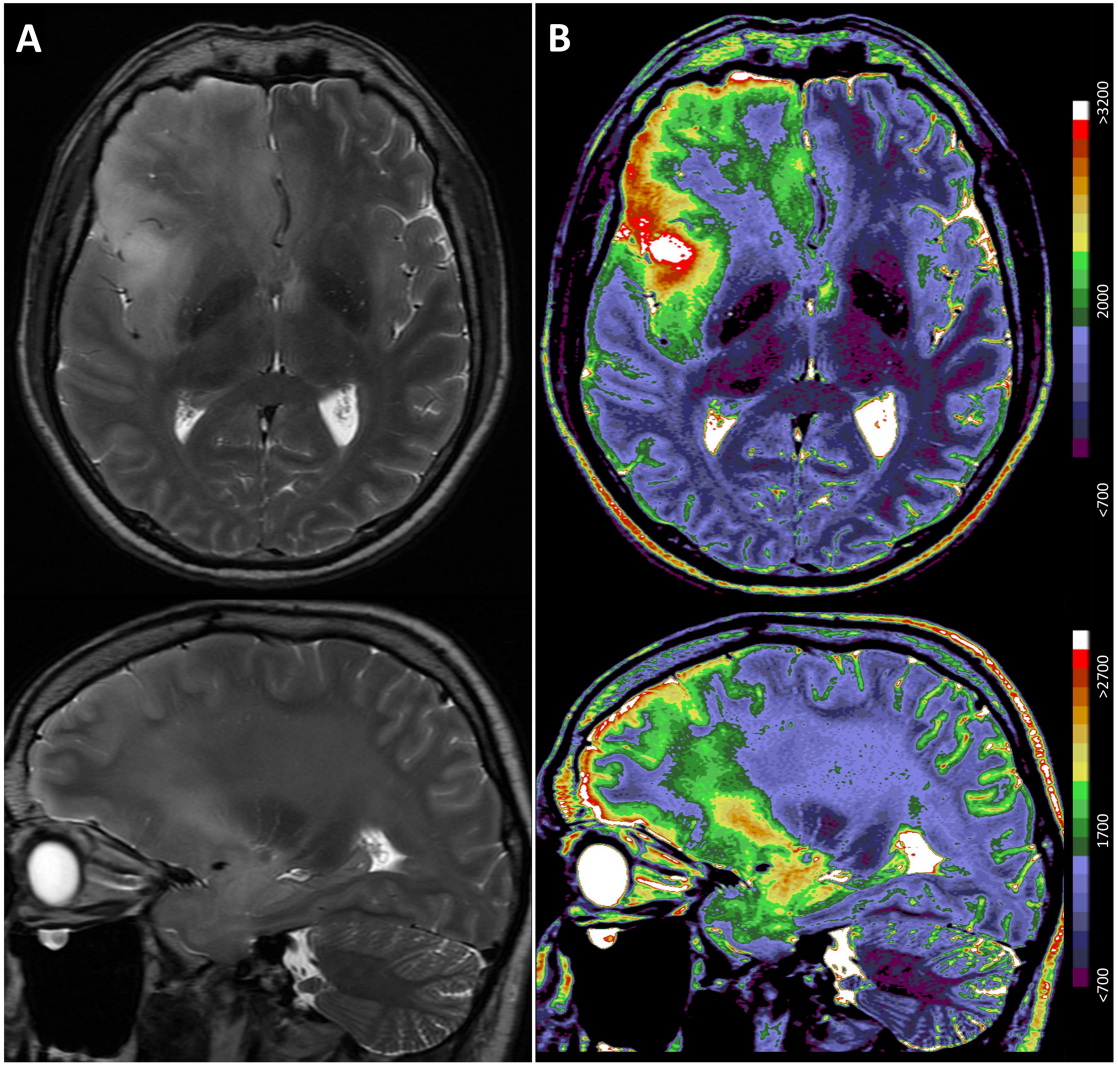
MRI T2-weighted scan (transversal and sagittal sections) of the presented patient, showing expansive lesion in the right-side medial frontal and temporal lobe with a massive oedema, affecting also corpus callosum and introducing midline shift, and a contrast-enhanced suprasellar expansion compressing the third ventricle and dislocating the optic chiasm. (A) Standard grayscale. (B) Complex colour scale for better visualisation, including the colour coding of T2-weighted values.
